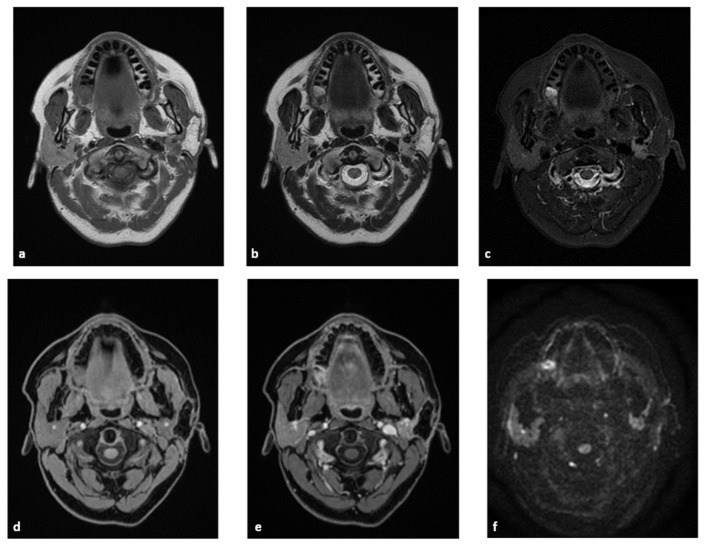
Female, 53 years old. First postoperative MR examination after partial parotidectomy with dermal fat graft. Fat appears hyperintense on both FSE T1-weighted (a) and T2-weighted (b) axial images and homogenously hypointense on STIR images (c). The fat graft shows no significant enhancement between pre- (d) and postcontrast (e) acquisitions and appears hypointense on DWI at b-1000 (f).
